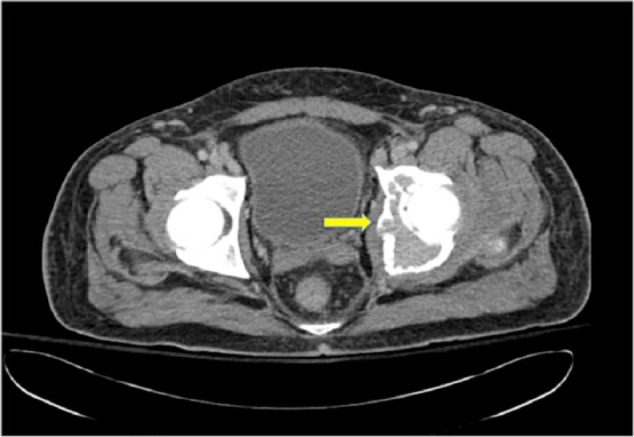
Transverse view of a pelvic CT scans showing a 9 × 6 cm osteolytic mass with sclerotic rim in the left acetabulum.
